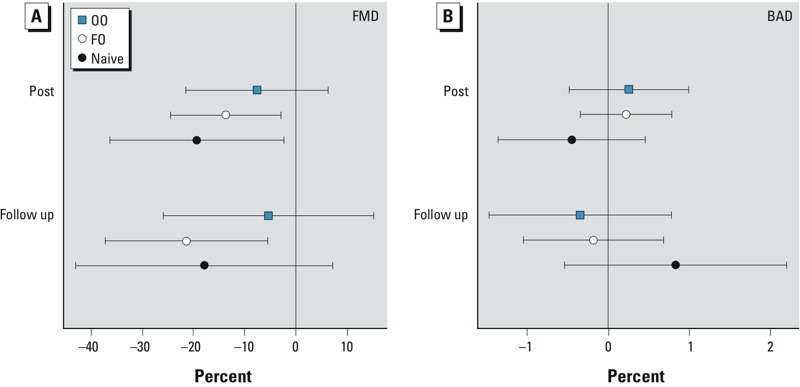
Effect of concentrated ambient particle (CAP) exposure on parameters of brachial artery ultrasound. Flow-mediated dilation (FMD; *A*) and baseline diameter (BAD; *B*) of the brachial artery were measured by ultrasound before, immediately after exposure to filtered-air and CAP (post), and again the next morning (follow-up) as described in “Methods.” Symbols represent differences between CAP and filtered-air exposure, expressed as average percent point differences per 100-μg/m^3^ increase in CAP, relative to the baseline; bars indicate 95% CIs.
